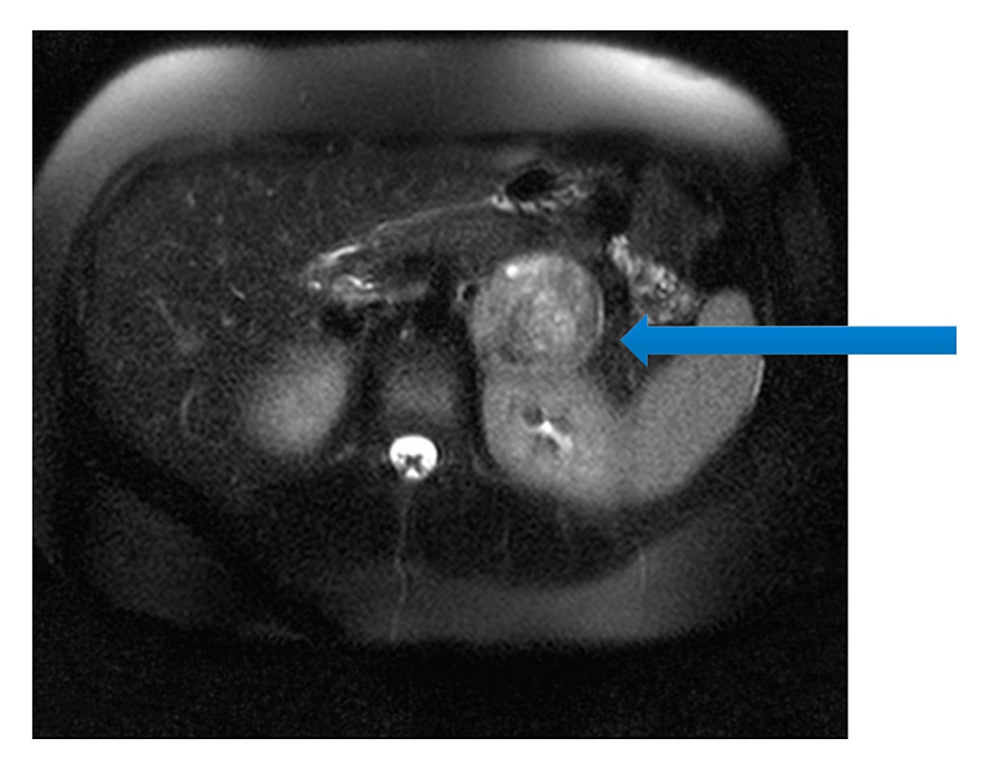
Magnetic resonance imaging of the abdomen in T2, showing the left mass corresponding to the paraganglioma (blue arrow)
